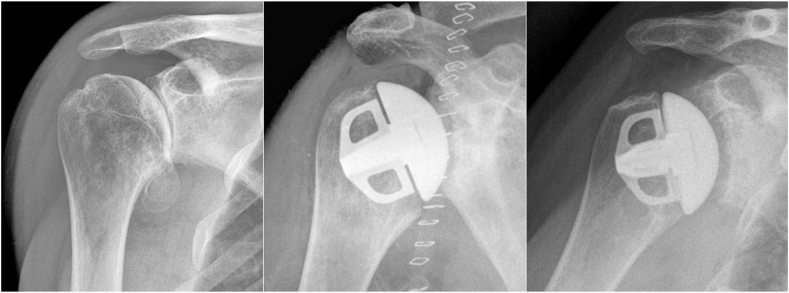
A typical aTSA case with the Affinis Short prosthesis. Radiographs taken preoperatively, immediately postoperatively, and at 10 years postoperatively. *aTSA*, anatomic total shoulder arthroplasty.
